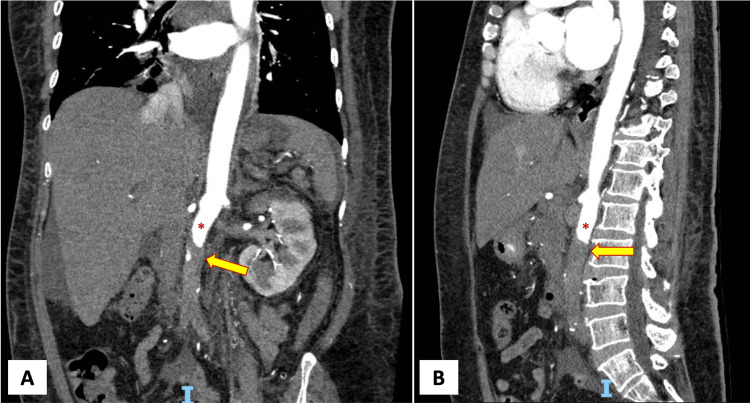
Computed tomography angiography demonstrating acute aortoiliac occlusion Coronal (A) and sagittal (B) views of CT angiography demonstrate contrast opacification of the abdominal aorta proximal to an abrupt cut-off at the L1-L2 level (yellow arrows), consistent with acute occlusion of the abdominal aorta. Red asterisks indicate the contrast-opacified abdominal aorta proximal to the occlusion. The complete CT angiography examination demonstrated associated bilateral common iliac artery occlusion.
